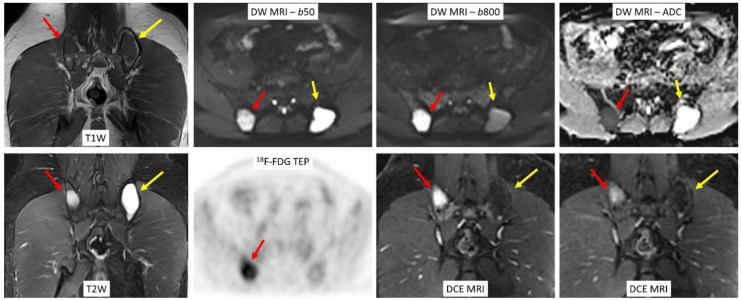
Coronal T1-weighted spin-echo and fat-suppressed T2-weighted images of the pelvis shows bilateral focal lesions in the right and left iliac bones, hypointense on T1- and hyperintense on T2-weighted images. Focal lesion on the right iliac bone (red arrows) appears to be metabolically active on transverse ^18^F-FDG PET image, has high signal intensity on both low and high *b* value DW images, higher ADC value than surrounding, and apparently normal bone marrow. Coronal DCE MR image shows an early and intense contrast-enhancement after contrast media administration, consistent with an active lesion. On the other hand, focal lesion on the left iliac bone (yellow arrows) appears to be metabolically inactive, and with no restriction of diffusion. Weak and thin linear and peripheral enhancement can be seen after contrast media administration, which is consistent with an inactive lesion.
